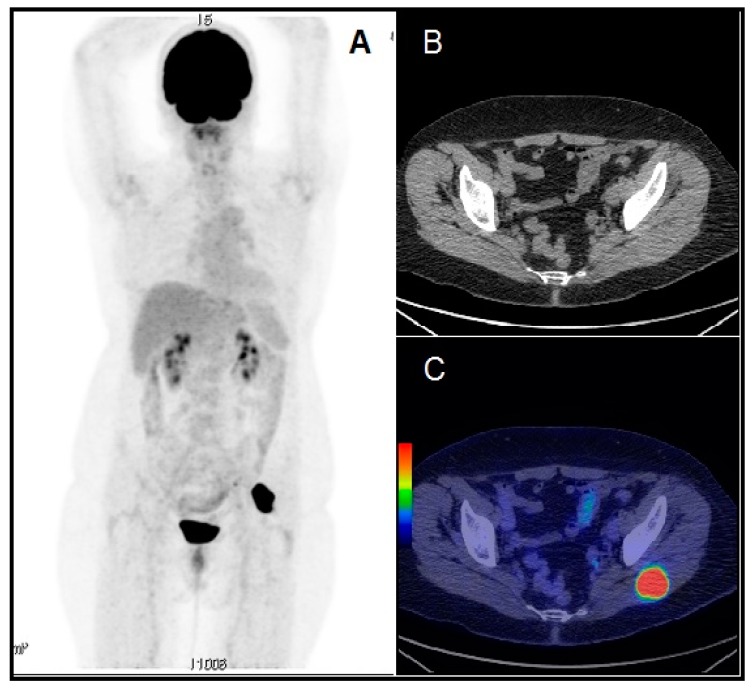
Whole body 18F-FDG PET/CT imaging revealed a right gluteal muscle tumor of 39 × 35 × 41 mm, with intense FDG uptake (SUV lbm max = 16.77) (A) 3D-MIP image. (B) CT axial image of the gluteal region. (C) PET/CT fusion image showing the intense uptake in the left gluteal muscle.
